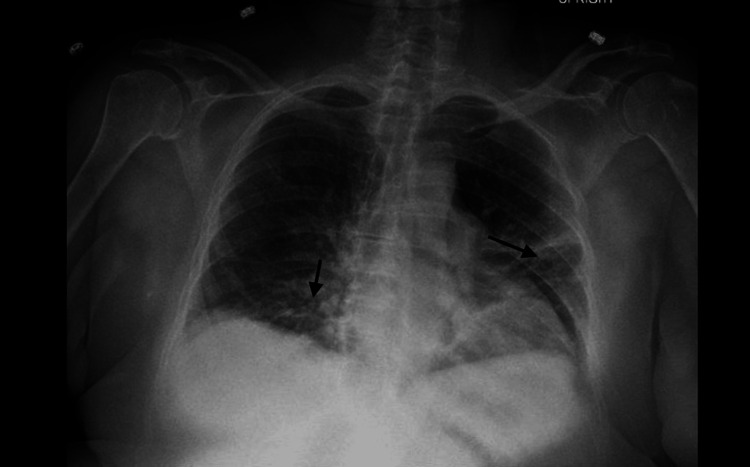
Chest radiograph showing an increase in atelectasis or infiltration within the middle and lower lung regions (arrows)
